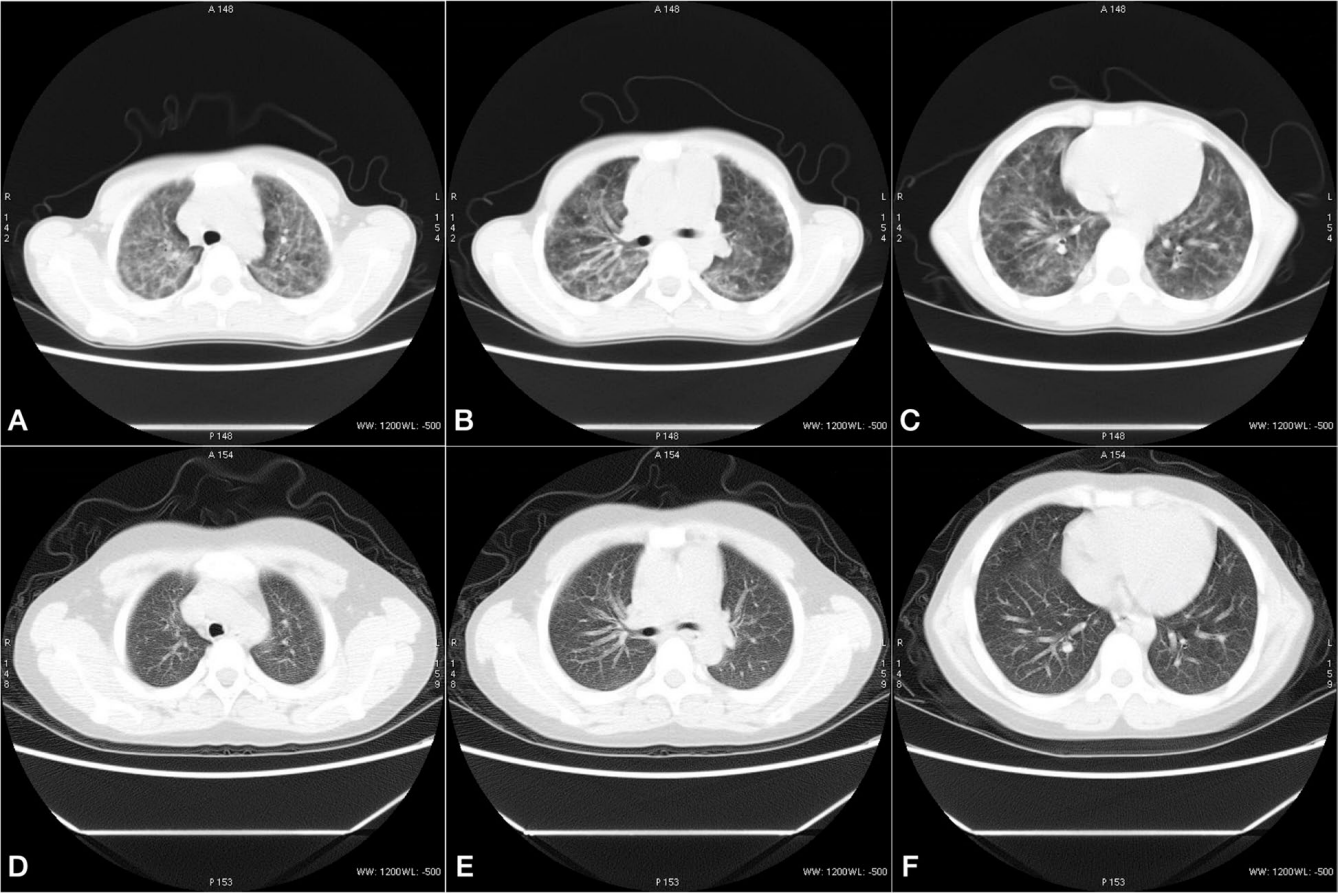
Comparison of chest HRCT images before (Nov. 09, 2018, A-C) and after (Jan.10, 2019, D-F) treatments for CAJDM-ILD. A-C shows diffused interstitial changes in both lungs, extensive ground glass shadows, fiber strips with grid syndrome, small mediastinal lymph nodes and partial pleural reaction on the right. D-F shows multiple clockwork shadows in both lungs, several tiny nodules can be seen in the right lung, and the upper left lung nodule has been absorbed
